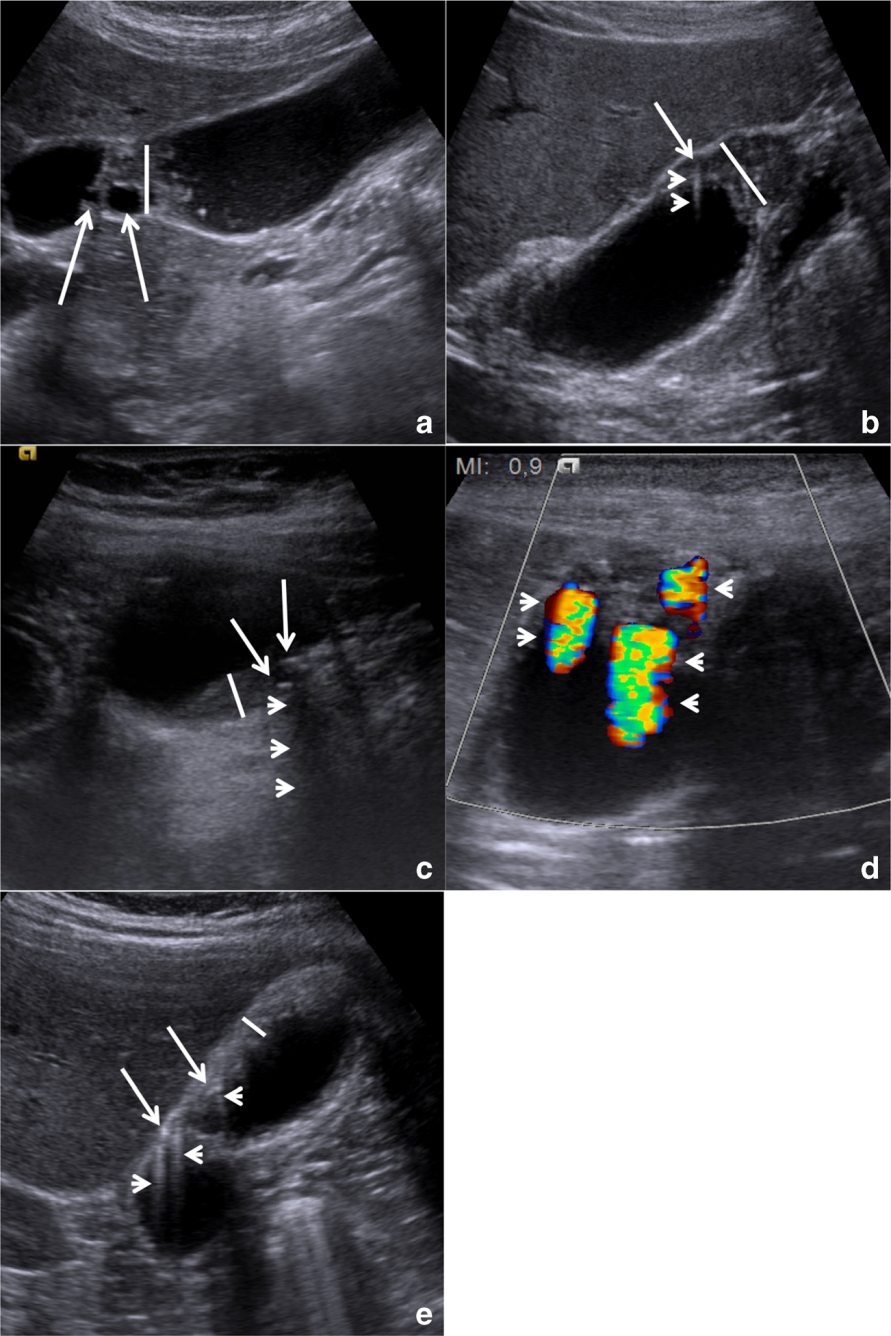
Gallbladder adenomyomatosis: typical US findings in annular type (a), fundal type (b and d), segmental type (c) and diffuse type (e). Gallbladder wall thickening (*line*) is always seen in gallbladder adenomyomatosis, but it is non-specific. On b-mode images, Rokitansky–Aschoff sinuses (*arrows*) typically appear anechoic (a), but they can also appear hyperechoic if cholesterine crystals or calcifications are present (b and c). Comet-tail reverberation artefacts (Figures *b* and *e*, *arrowheads*) or acoustic shadowing (c, *arrowheads*) are usually observed profoundly in RAS. On colour Doppler images (d), twinkling artefacts (*arrowheads*) may be observed profoundly in RAS
